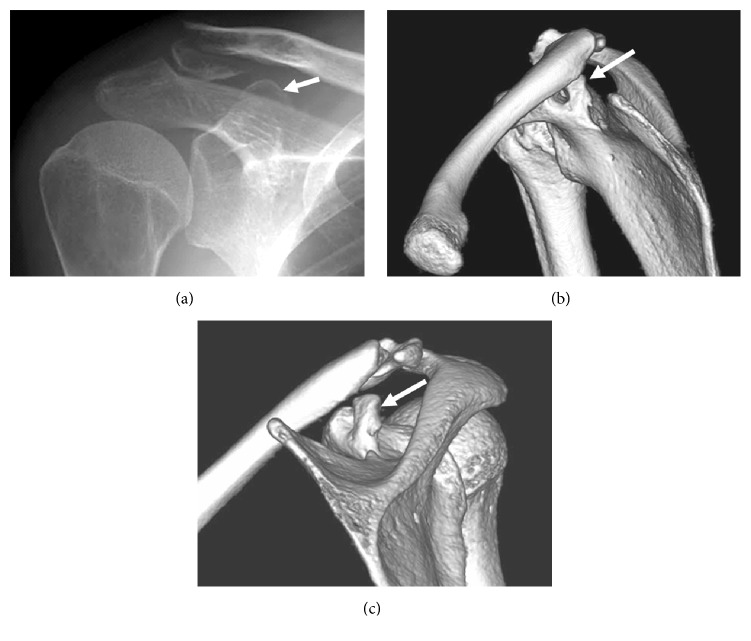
Imaging of Case  3. (a) Radiogram taken at the first visit revealing distal clavicular fracture (type 2b according to Craig's classification) and avulsion fracture of the posterior side of the coracoid process (arrow). (b and c) Three-dimensional computed tomography taken 1 year after the injury demonstrating nonunion of the lateral clavicular fracture and the malunited coracoid fragment migrating posterosuperiorly (arrow).
